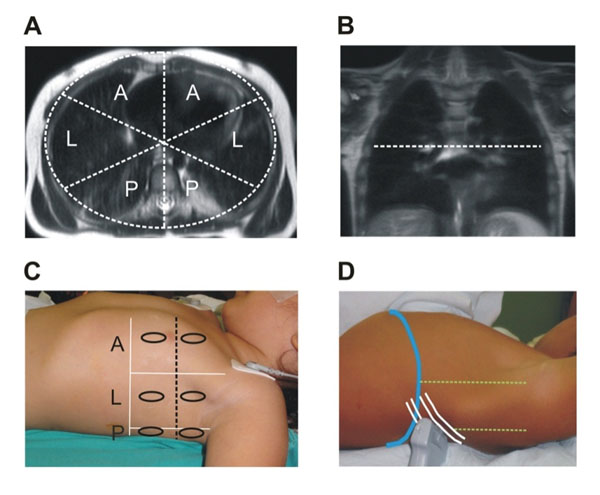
Chest segmentation of MRI-LUS image. A) The axial MRI cuts were segmented in anterior (A), lateral (L) and posterior (P) regions in both lungs. B) The coronal MRI view was used to divide the lungs in superior and inferior lungs regions. C) The LUS assessment followed similar chest regions than MRI images. The dotted black axial line separated the lungs in superior and inferior portions. Ovals depicted where the probe is placed in the classical LUS approach: mid-clavicular, lateral and posterior axillary lines. D) The posterior regions were also assessed by the inferior postero-basal LUS view.
